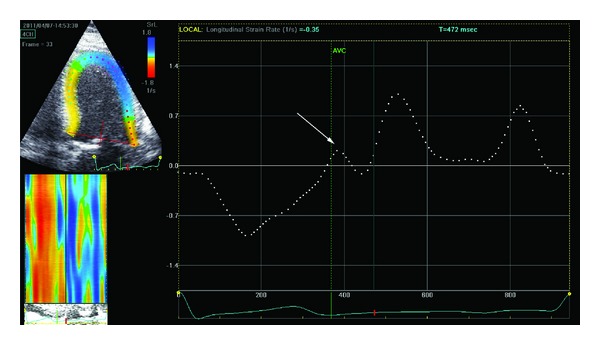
Evaluation of peak strain rate during left ventricular isovolumetric relaxation period SR_IVR_ from the apical 4-chamber view. First, a region of interest which includes the entire left ventricular wall is obtained (left-upper panel). The software consequently displays the changes in longitudinal strain rate over the cardiac cycle (white dotted line). Left ventricular isovolumetric relaxation occurs in diastole, starting immediately after closure of the aortic valve and terminating with the beginning of the diastolic early (*E*) wave. The peak strain rate value during the isovolumetric relaxation period (SR_IVR_) is consequently measured (indicated by the white arrow). The green dotted line indicates the timing of the aortic valve closure (AVC).
